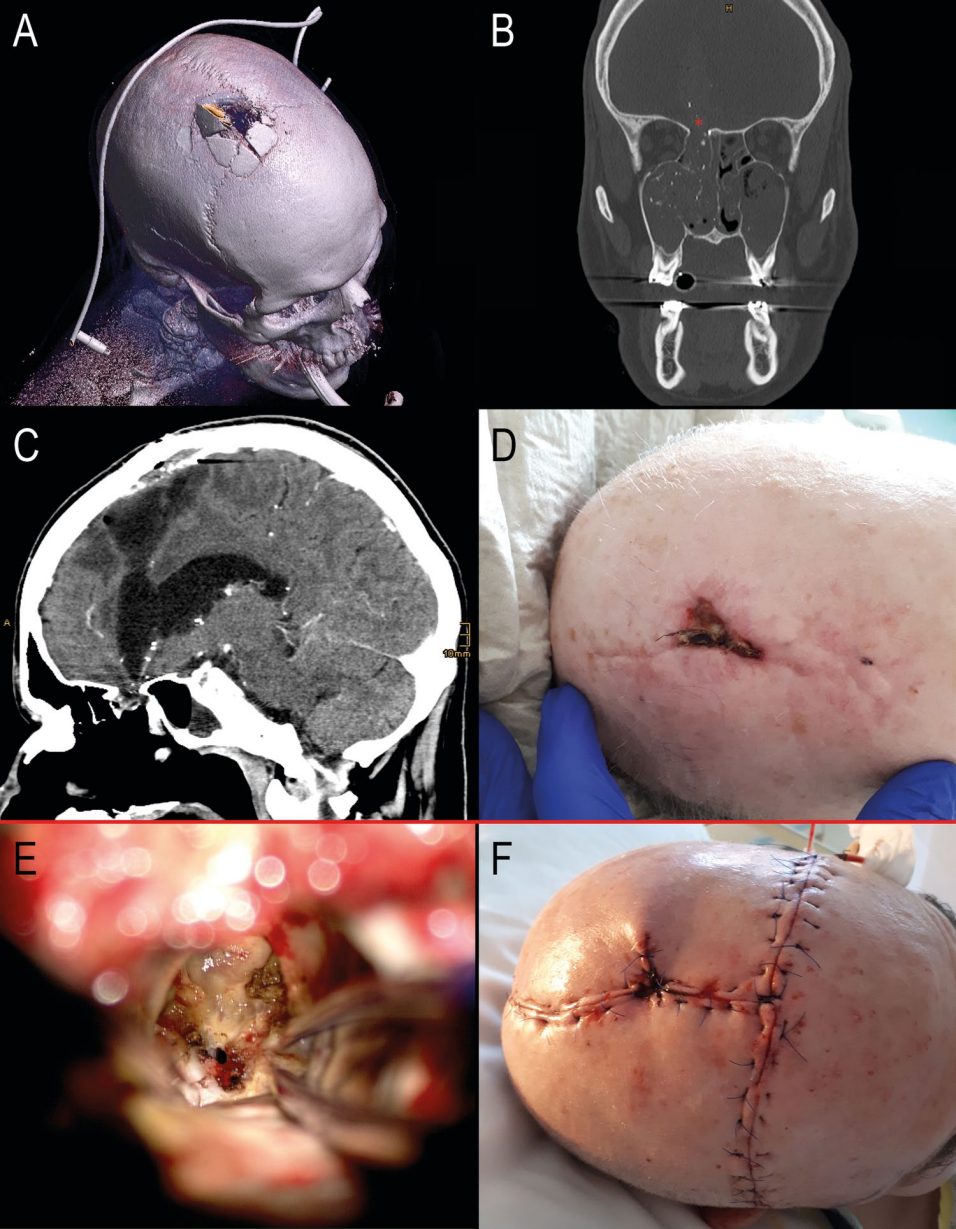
Patient 13, a 60-year-old male, sustained a self-inflicted cranial gunshot wound with the bullet entering at the base of the frontal region and exiting through the upper aspect of the frontal bone. Initially managed at an external institution, his treatment included dural closure and bone coverage with a heterologous bone graft. One month post-injury, he was transferred to our facility due to elevated infection markers, rhinoliquorrhea, and a significant brain abscess. Postoperatively, the patient showed good recovery. At the last clinical follow-up 21 months later, the patient now resides in a care facility, presenting no focal neurological deficits but continues to experience recurrent aggressive outbursts and emotional instability, due to preexisting psychiatric conditions and acquired frontal lobe damage. A Initial post-traumatic CT scan showing a right parasagittal bone defect at the bullet exit site. B Right frontobasal bone defect at the bullet entry site. C Intracerebral abscess formation extending from the frontobasal region to the high frontal area. D Preoperative view showing a 3.4 cm² frontal wound dehiscence with purulent secretion. E Intraoperative view of the frontobasal bone defect, accessed through the abscess from the high frontal region without necessitating a second craniotomy. Dural defects were covered with fascia lata. F Postoperative day 1, showing large skin incisions involving a preexisting scar
